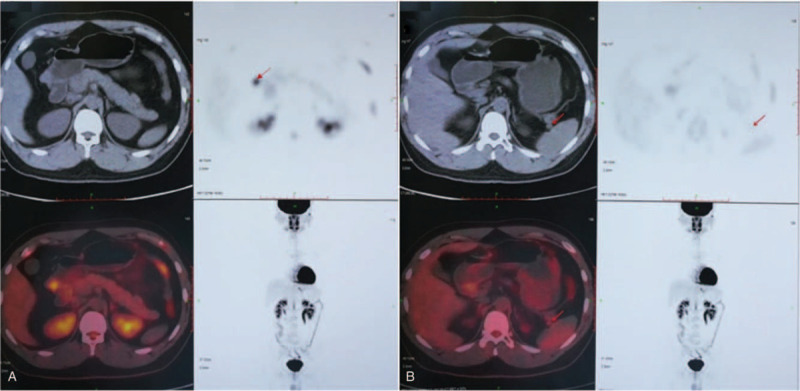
^18^F-FDG PET/CT showed abnormal concentration of tracer in the lesion on the right side of the gastric antrum (A). The nodule in the tail of the pancreas showed no abnormal concentration of tracer (B).
